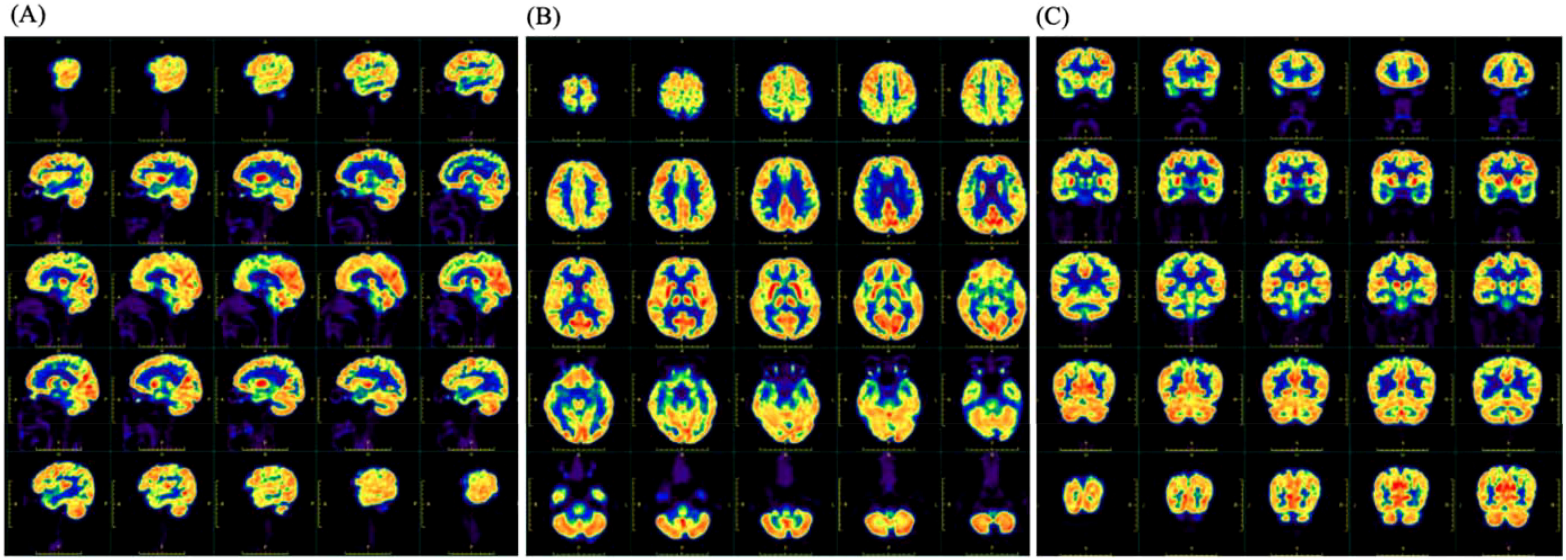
The image of ^18^F-FDG PET showed slightly decreased metabolism in the right parietal lobe, bilateral parts of the frontal lobe, left anterior cingulate gyrus, and right parietotemporal junction areas. (A) The sagittal position. (B) The transverse position. (C) The coronal position.
